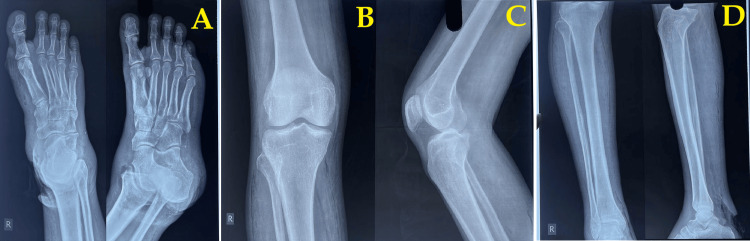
X-ray image of the infected foot A: Anteroposterior view. B: Anterior view. C: Lateral view. D: Tibia-lateral and anterior view.
